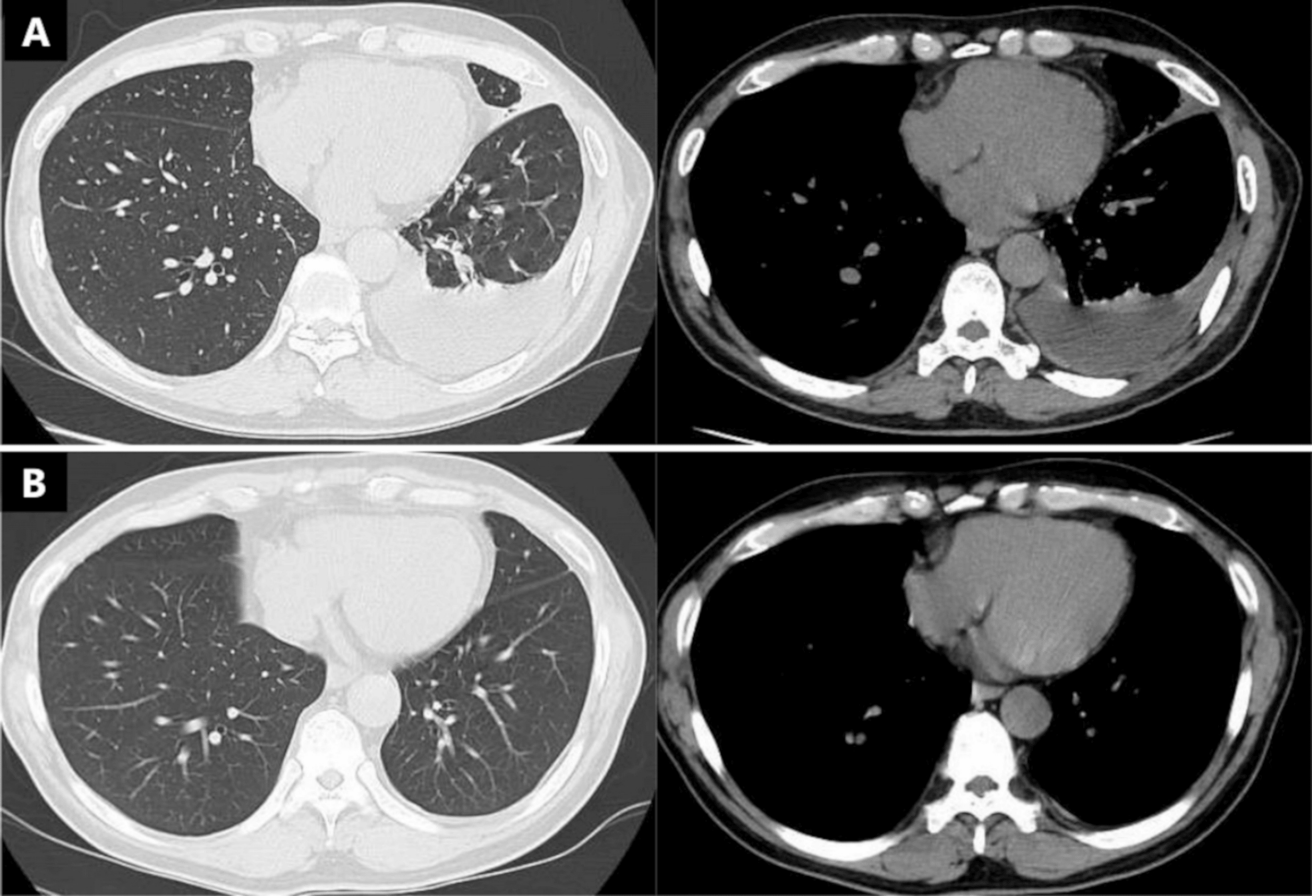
Changes in RPE observed via chest computed tomography (CT). (A) Left-sided RPE at initial presentation. (B) Resolution of RPE three months after sarilumab initiation. RPE, rheumatoid pleural effusion
